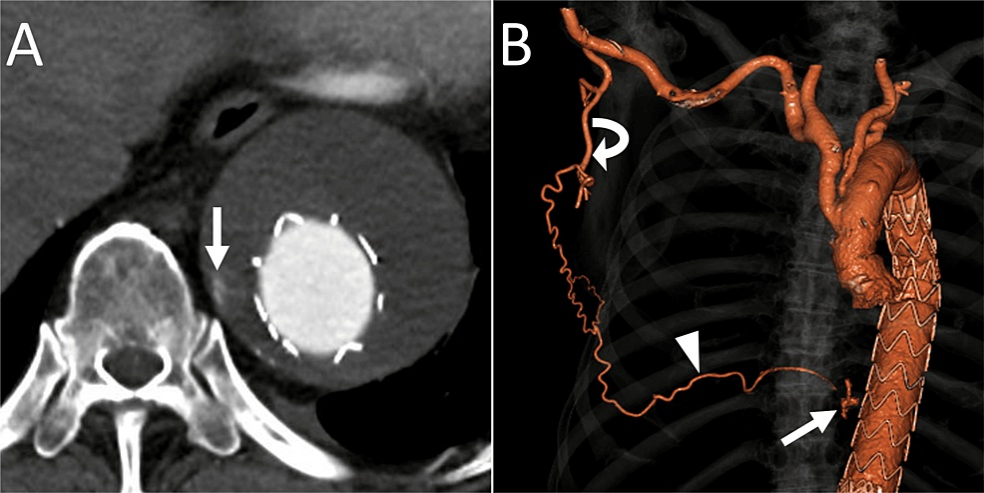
Contrast-enhanced CT before transarterial embolization for a type 2 endoleak. (A) Axial arterial phase contrast-enhanced CT image of the lower thorax showing an endoleak (arrow). (B) Anterior view of three-dimensional CT showing a type 2 endoleak (arrow) through the thoracodorsal artery (curved arrow) and the ninth intercostal artery (arrowhead). CT: computed tomography
